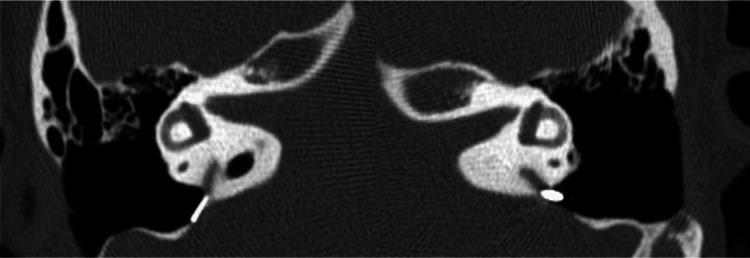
Postoperative CT. Postoperatively, the clips have been maintained without problems.
